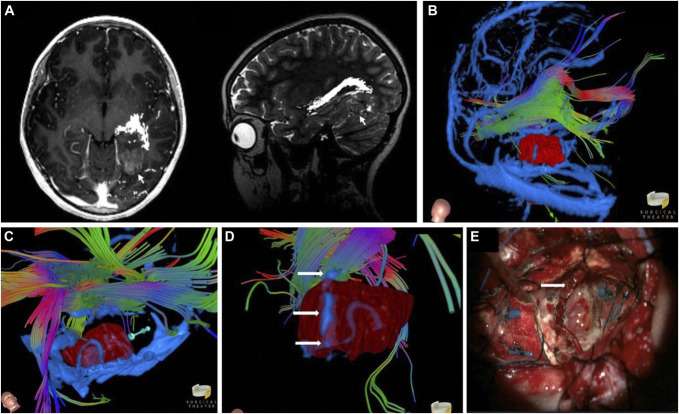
Left temporal neuroepithelial tumor of the young (PLNTY). A, Preoperative axial T1 and sagittal T2-weighted magnetic resonance B. Virtual reality 3-dimensional view from a posterior-lateral approach with the proposed operative trajectory to lesion (red). The optic radiations travel superiorly away from the lesion. C, “Bottom-up” view reveals a serpiginous vessel along the deepest surface of the lesion with the proposed posterior trajectory (turquoise arrow). D, High-magnification view from a lateral view shows a possible *en passage* vessel (arrows) traveling through the lesion and toward the optic radiations. The deep serpiginous vessel is seen in silhouette through a partially translucent lesion. E, Intraoperative image reveals a fully dissected *en passage* vessel (arrow) and the deep serpiginous vessel (arrowheads).
